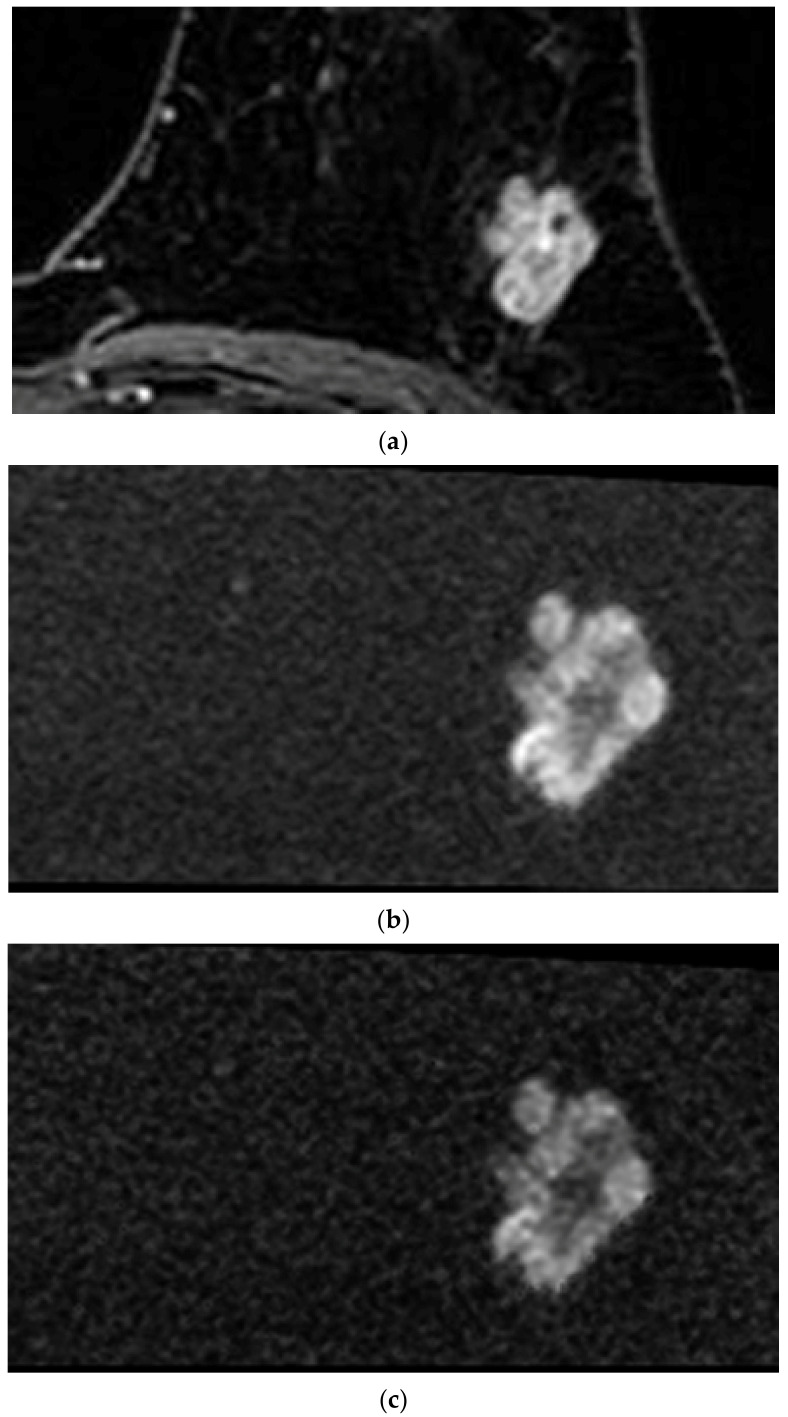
A 63-year old woman who had medullary carcinoma in her right breast. Axial contrast-enhanced T1-weighted fat-suppressed MRI (a) shows a 19 × 25 mm-sized enhancing mass with irregular shape, irregular margin, and heterogeneous and marked enhancement in the left 2 o’clock direction. rFOVA-1000 ^a^ (b) and rFOVS-1500 ^b^ (c) reveals the well-delineated index tumor, and the irregularity of the shape and the margin of the index tumor is also well depicted. ^a^ rFOVA-1000: acquired reduced field-of-view diffusion-weighted images with *b*-value 1000 s/mm^2^. ^b^ rFOVS-1500: synthetic reduced field-of-view diffusion-weighted images with *b*-value 1500 s/mm^2^.
